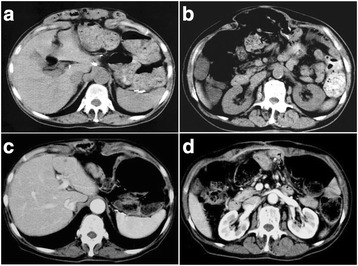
CT scan before and after surgery. a, b A large hernia with prolapsed small intestine was observed before surgery. c, d Mesh sheet covering the hernial orifice
